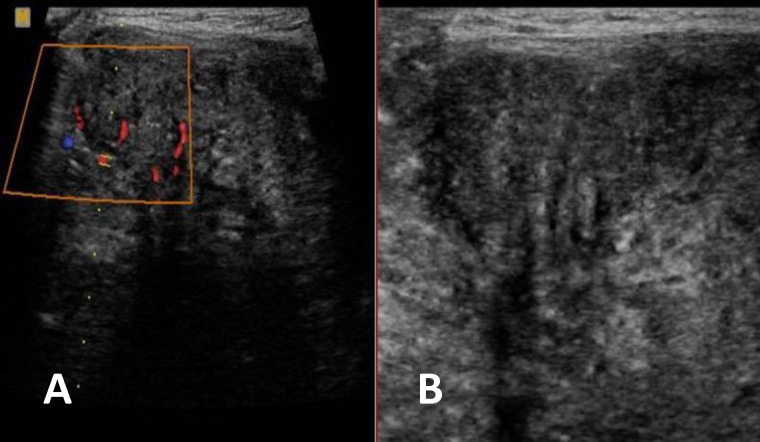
Cervical US scan in axial plane through the left Submandibular region (A,and B)shows a heteroechoic solid mass containing scattered hyperechoic foci(arrow) . Color Doppler imaging in the same axial scanning plane (A) shows vascularization within the mass
